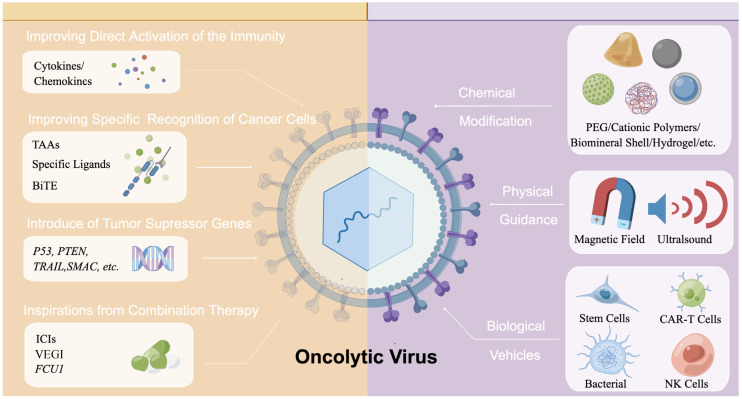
Strategies for improving oncolytic virotherapy. Strategies are divided into two main categories: improvements of genome engineering and of the delivery system. In terms of the genome engineering (left), various functional elements can be designed into the genome of oncolytic virus, including those can activate the immune system directly, specific recognition of cancer cells, tumor-suppressor genes, and inspirations from other therapies. In terms of the delivery system (right), multiple nanomaterials could be used as chemical modifications to the capsule of viruses. In addition, physical technology such as magnetic fields and ultrasound could also help in the guidance of oncolytic viruses. Stem cells, CAR-T cells, and even bacteria could be used as vehicles to transport oncolytic viruses. TAAs: Tumor-associated antigens; BiTE: Bispecific T cell engager; ICIs: Immune checkpoint inhibitors; VEGI: vascular endothelial cell growth inhibitor; PEG: poly(ethylene) glycol; CAR: chimeric antigen receptor; NK cells: natural killer cells. The figure is drawn by FigDraw.
